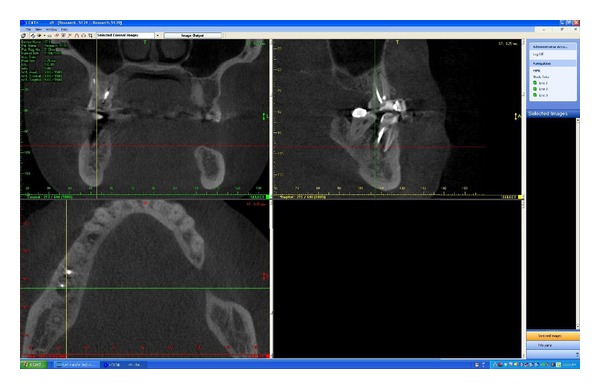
Cone Beam CT image of molar tooth as viewed by observers in the study.
